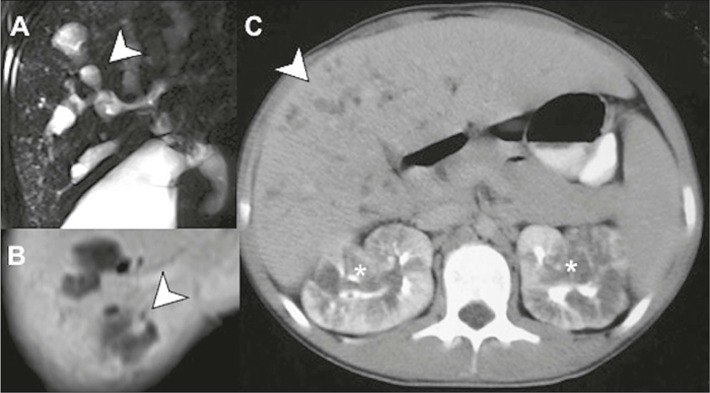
Caroli’s disease. MRI cholangiography with maximum intensity
